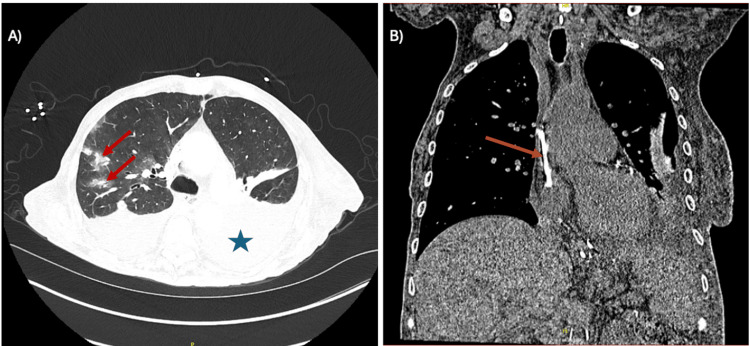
CT imaging of bilateral septic emboli and the retained intravascular catheter (A) Axial chest CT demonstrating bilateral multifocal ground-glass opacities and nodular consolidations, most prominent in the right lung (red arrows), consistent with septic emboli. Also noted are bilateral pleural effusions, more pronounced on the left (blue star). (B) Retained catheter fragment extending from the left innominate vein to the cavoatrial junction (orange arrow).
